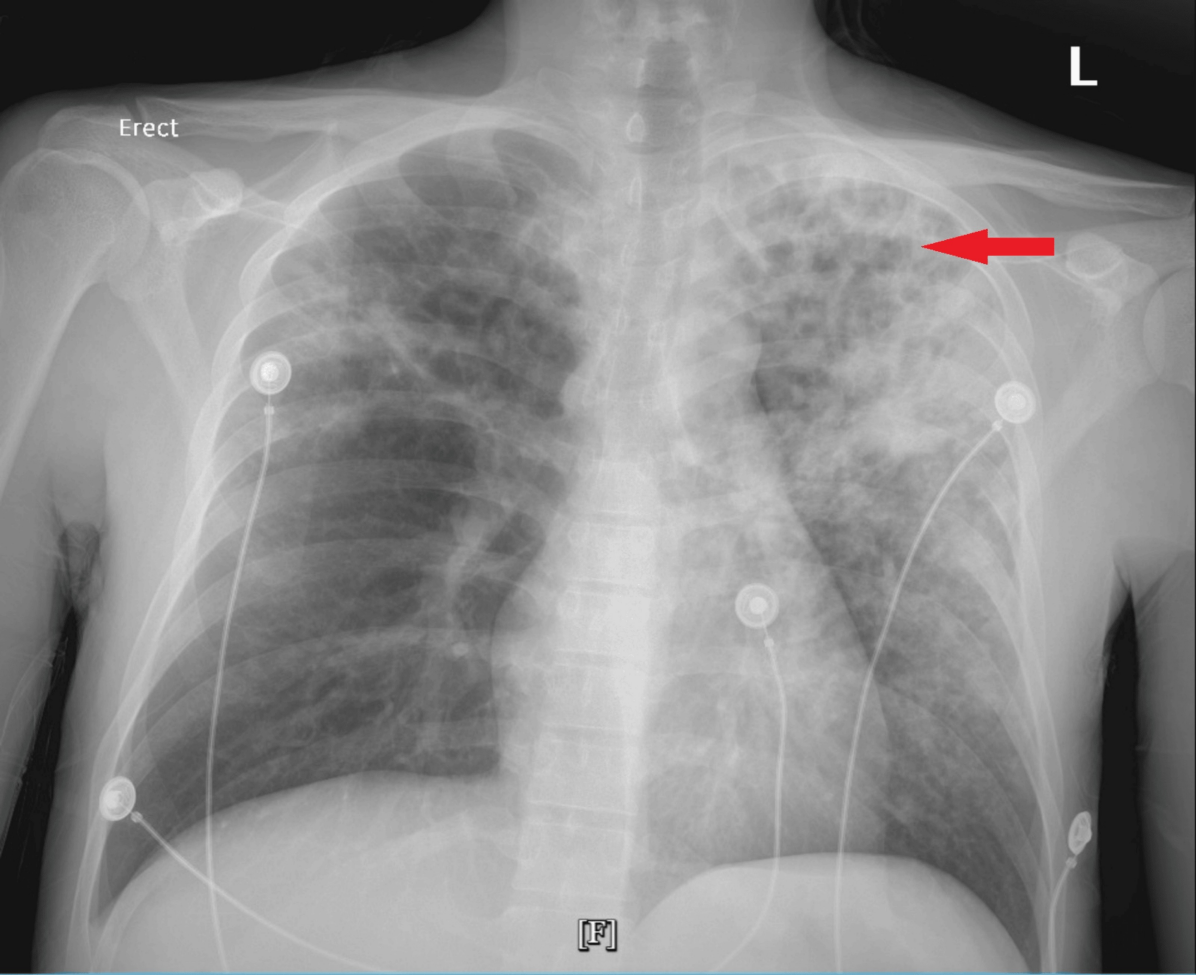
Chest x-ray showing patchy consolidation with cavitation and fibrosis in the left upper lung zone (red arrow)
